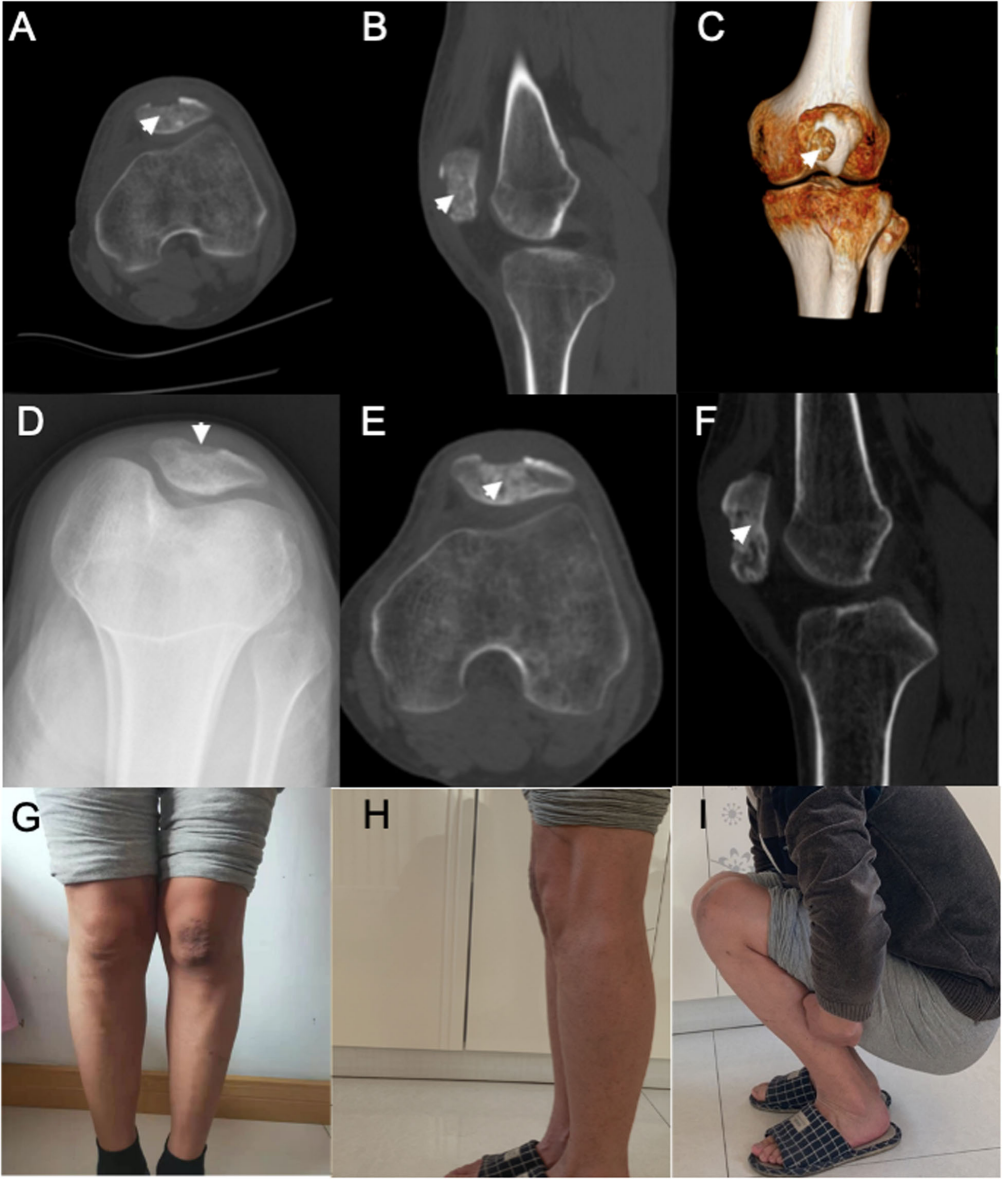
Postoperative imaging data (a-c). The fenestration for the focus was found to be reasonable in the postoperative reconstructed, axial, and sagittal CT, and patella-reconstructed CT (d-f). X-rays and CT scans showed that the grafted bone had been partly incorporated with the host bone 2 months after the operation (g-i). Two months after the operation, the range of motion of the knee joint was normal
